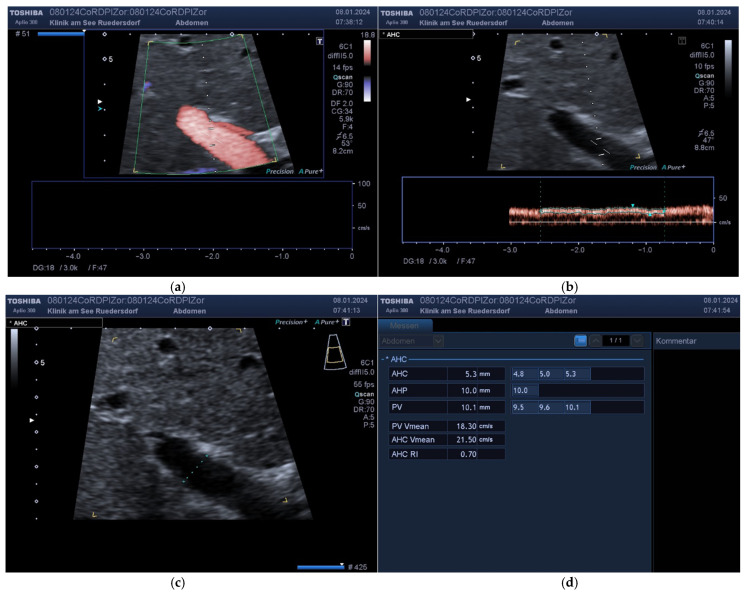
Measurement of a PV. (a) Color. (b) Doppler with a window corresponding to the diameter. (c) Determination of the diameter. (d) Documentation of the results in the preset.
